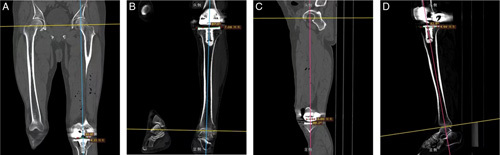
Postoperative computed tomography scans. (a) Measurement of the coronal femoral component angle. (b) Measurement of the coronal tibial component angle. (c) Measurement of the sagittal femoral component angle. (d) Measurement of the sagittal tibial component angle.
